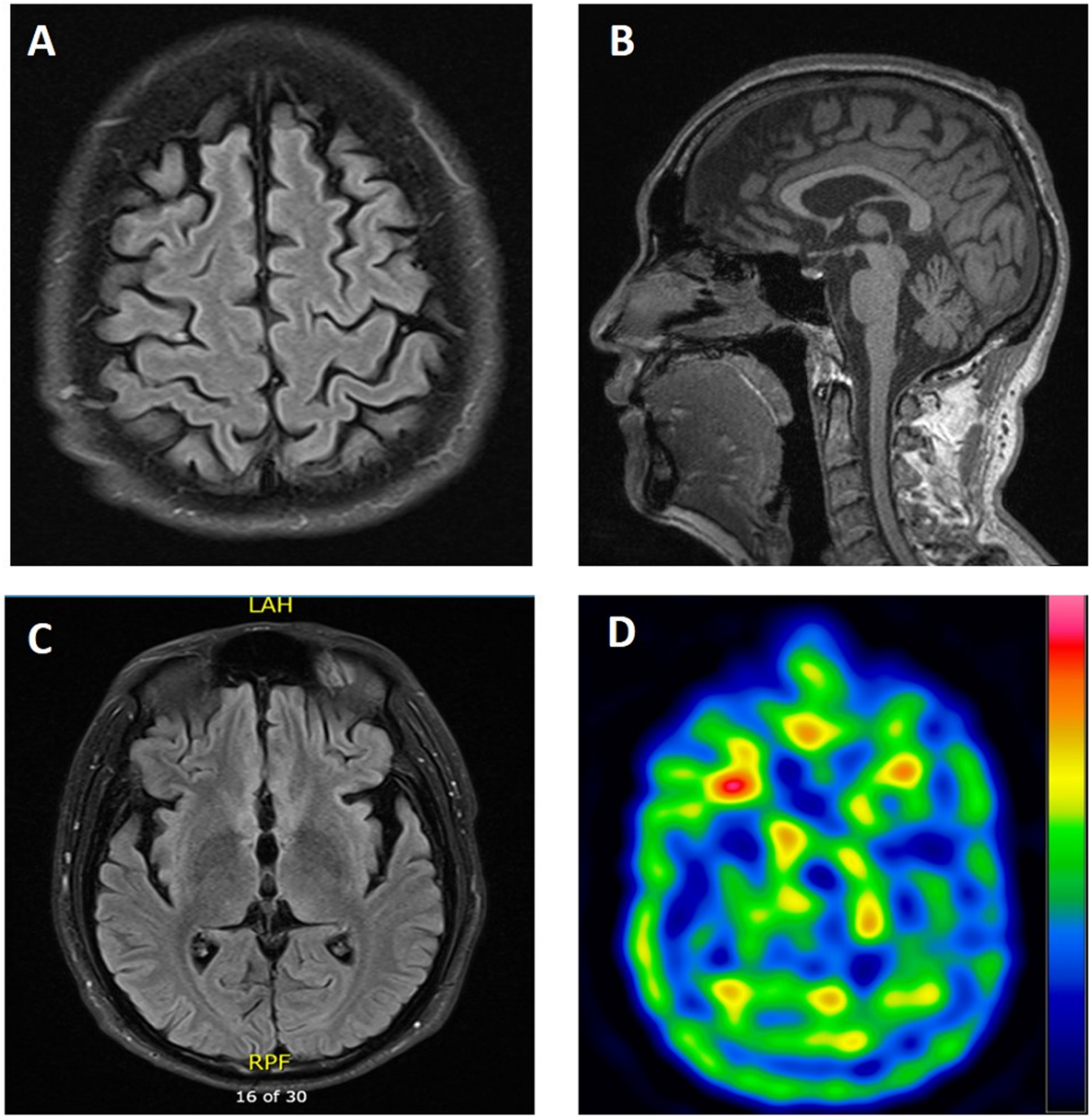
Brain imaging findings in the first index patient. (a, b, c) Brain MRIs with T2 Fluid Attenuated inversion recovery (FLAIR) sequence show (a) diffuse atrophy in the cortex and cerebellum and (b) cerebellar atrophy, but (c) no abnormal signs of iron accumulation in the globus pallidus. (d) A Tc-99 m TRODAT scan reveals reduced dopamine transporter activity in the bilateral basal ganglia
